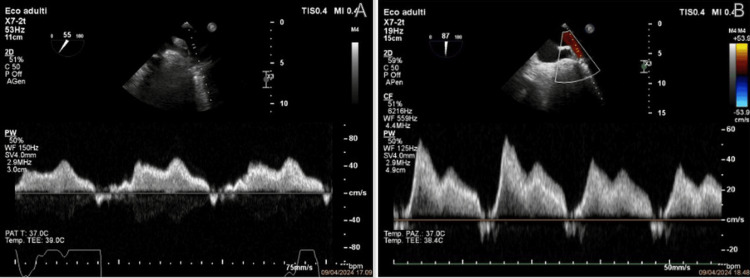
Pulmonary vein Doppler images (A) Pulmonary vein Doppler before MitraClip® showing systolic flow reversal. (B) Pulmonary vein Doppler after clip deployment demonstrating restoration of systolic-dominant flow
